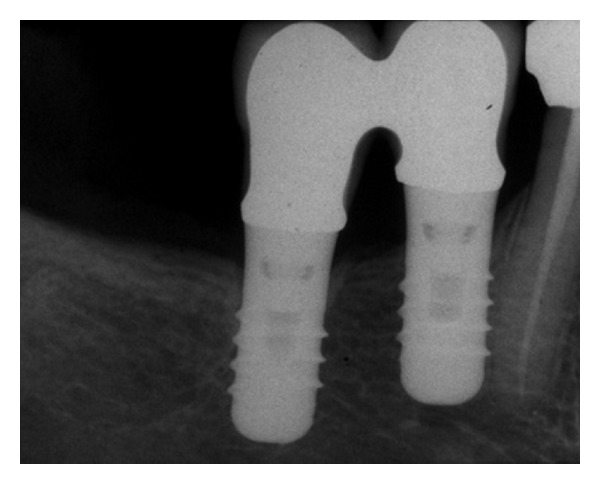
Partial fixed prosthesis supported by short implants (4.1 × 8 mm): 14 years loading. Periapical radiograph.
